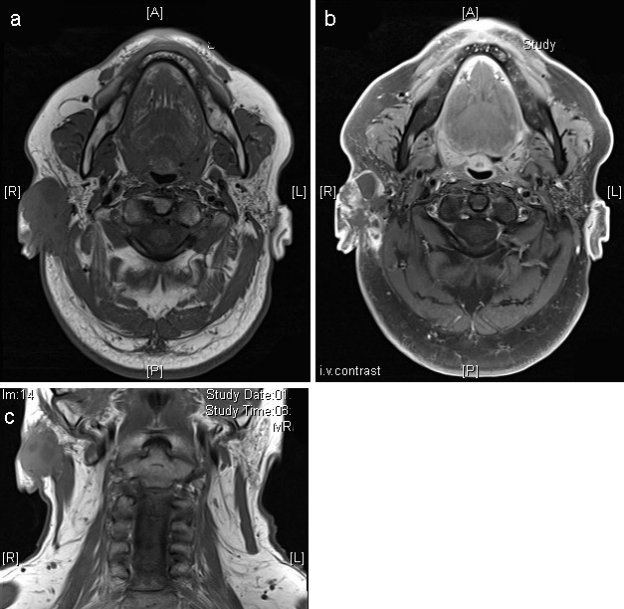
a: Preoperative magnetic resonance imaging (MRI) – axial section (tra tse t1). The hypointense tumor represents a mass of 47.5x43x34.6 mm that infiltrates the caudal meatus acusticus externus and the parotid gland, attaining up to the sternocleidomastoid and nuchal muscles (rT4N1M0). b: Preoperative MRI – axial section (tra tse t1 with contrast agent). The tumor is characterized by inhomogeneous cysteiform contrast agent uptake. c: Preoperative MRI – coronal section (tse t1)
